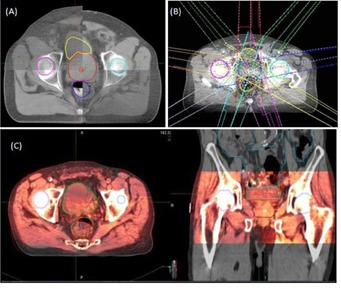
Soft tissue alignment (a) before treatment; (b) beam arrangement on daily CBCT; (c) rigid fusion of planning CT & daily CBCT to transfer contours.
